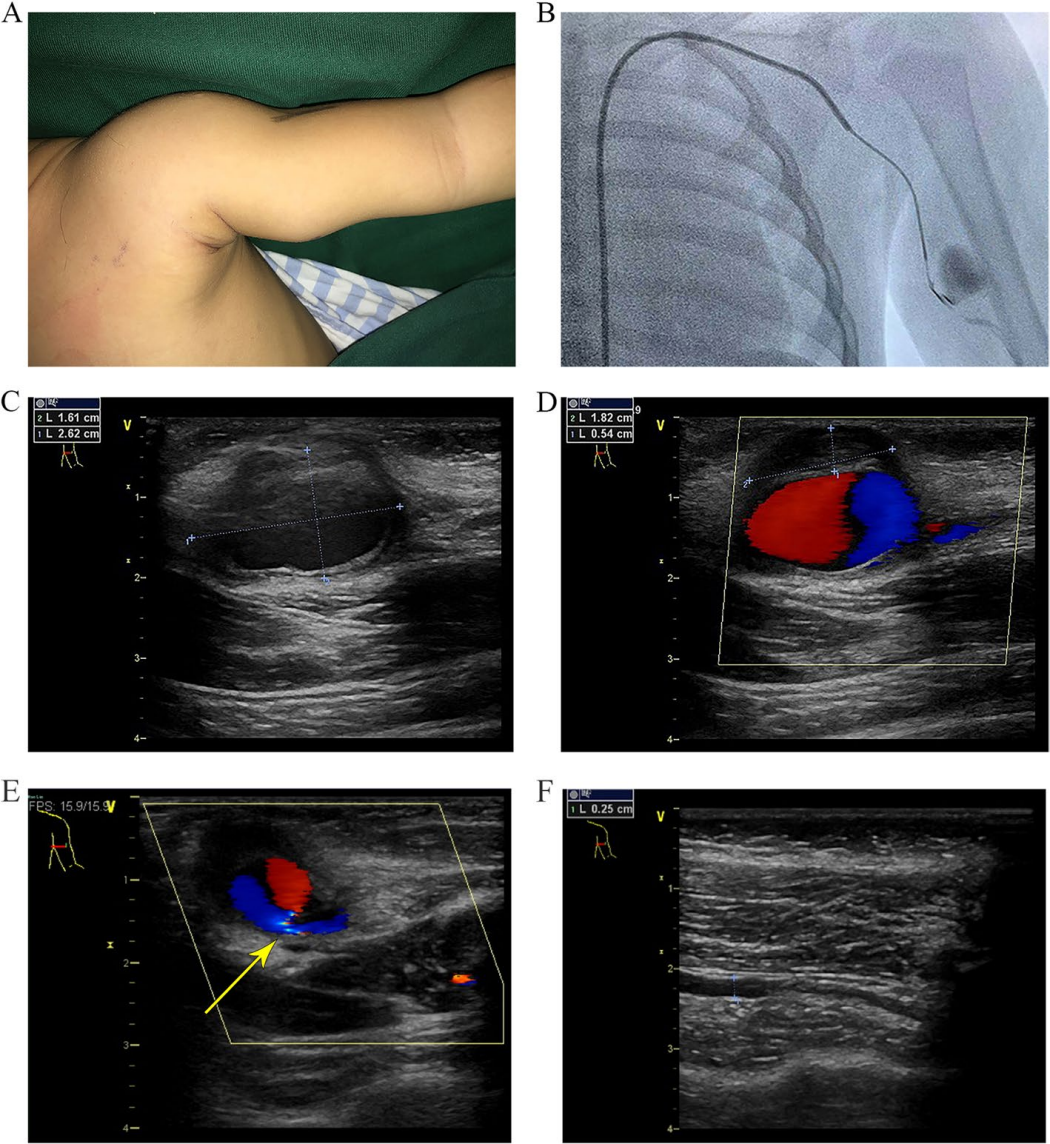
Preoperative examination of the patient. A A mass can be seen under the skin in the medial left arm. B Digital subtraction angiography revealed an eccentric aneurysm in the brachial artery. C Doppler ultrasonography revealed a cystic mass measuring 2.62 cm × 1.61 cm. D Swirling flow pattern in the mass was confirmed with thrombus adherent to the wall of the mass. E Preoperative ultrasound measurement of pseudoaneurysm neck is very thin, ~ 0.2 cm in length. F Preoperative ultrasound measurement of normal segment brachial artery diameter—0.25 cm
